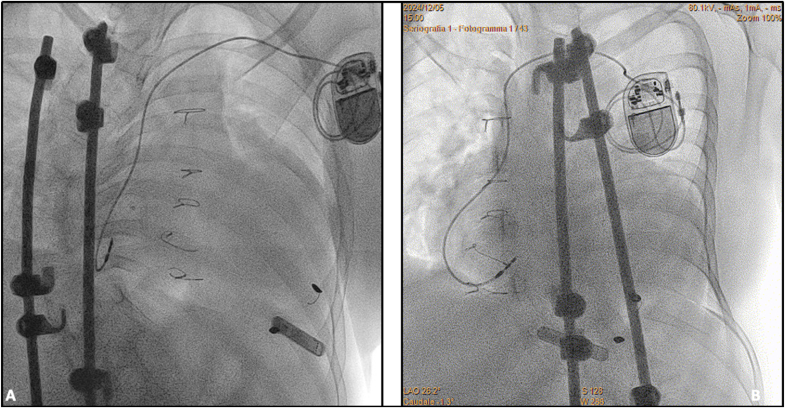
the X-ray image in right anterior oblique (RAO) view (A) and left anterior oblique (LAO) view showing the final position of the atrial lead.
